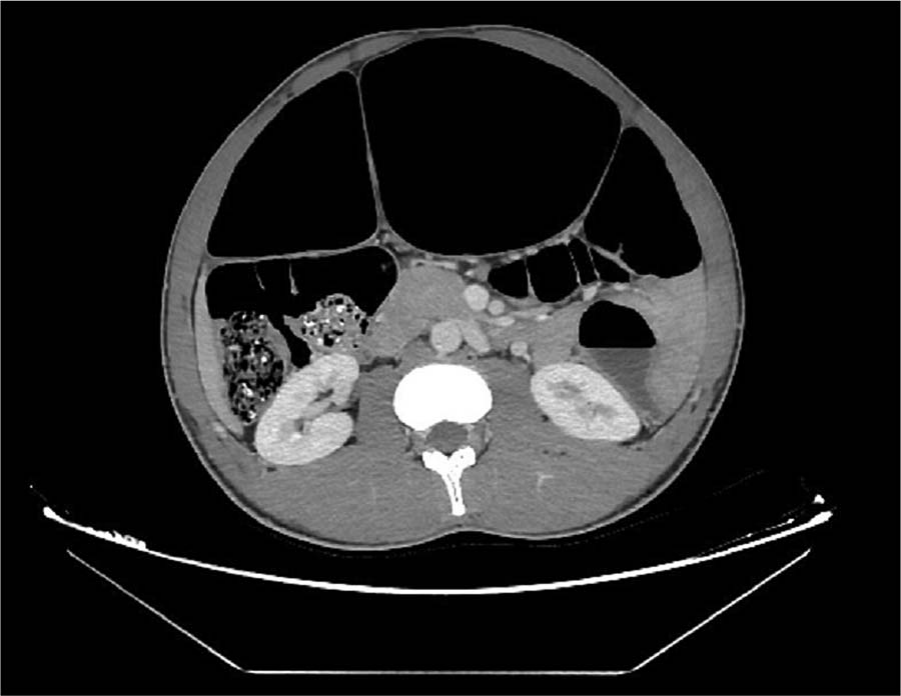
Axial abdominal CT scan revealed dilated gas-filled loops with lack of the haustration.
